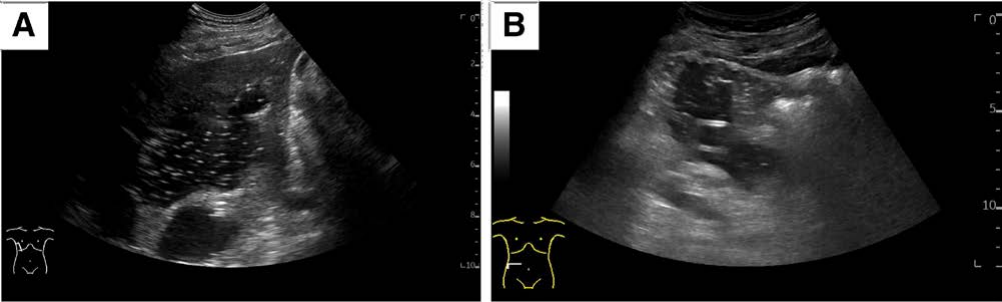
US-guided sclerotherapy for giant hepatic and renal cyst. (A) Sclerotherapy with an injection of medical anhydrous ethanol after evacuation of hepatic cystic fluid. (B) Sclerotherapy using the injection of medical anhydrous ethanol following the drainage of renal cystic fluid. US = ultrasound.
